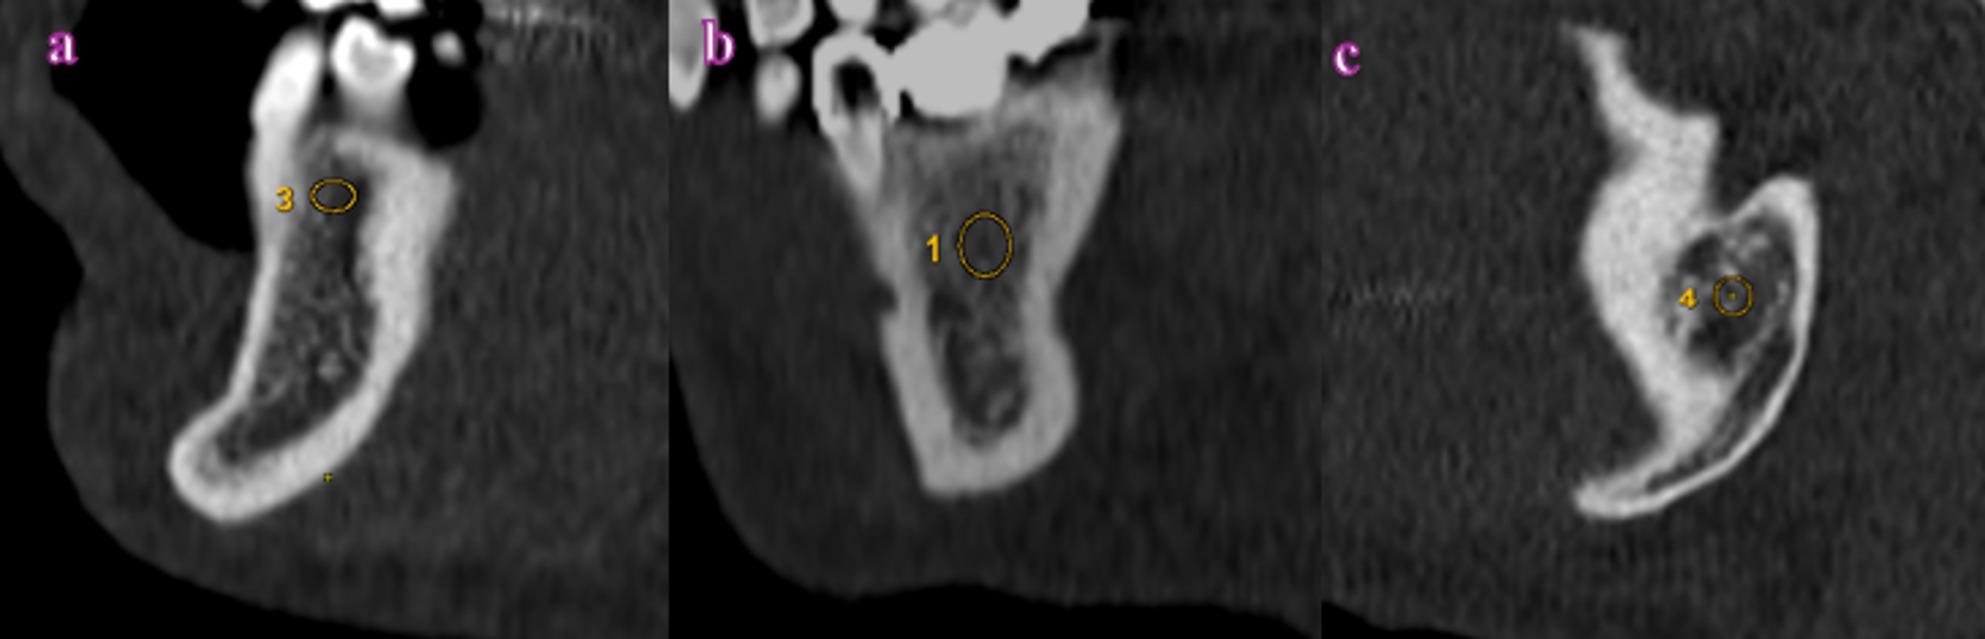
Densitometric measurements from CT images (on sagittal slices): (a) Interdental region between teeth 3 and 4 (214 HU), (b) Area inferior to the apex of tooth #6 (317 HU), (c) Mandibular angle region (405 HU). (Numerical labels visible within the ROIs are software-generated display markers and have no analytical significance)
